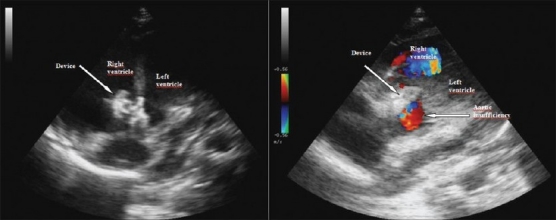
Closing of VSD using Amplatzer duct occluder 2 and checking of the aortic insufficiency shortly after releasing the device on transthoracic echocardiographic imaging
